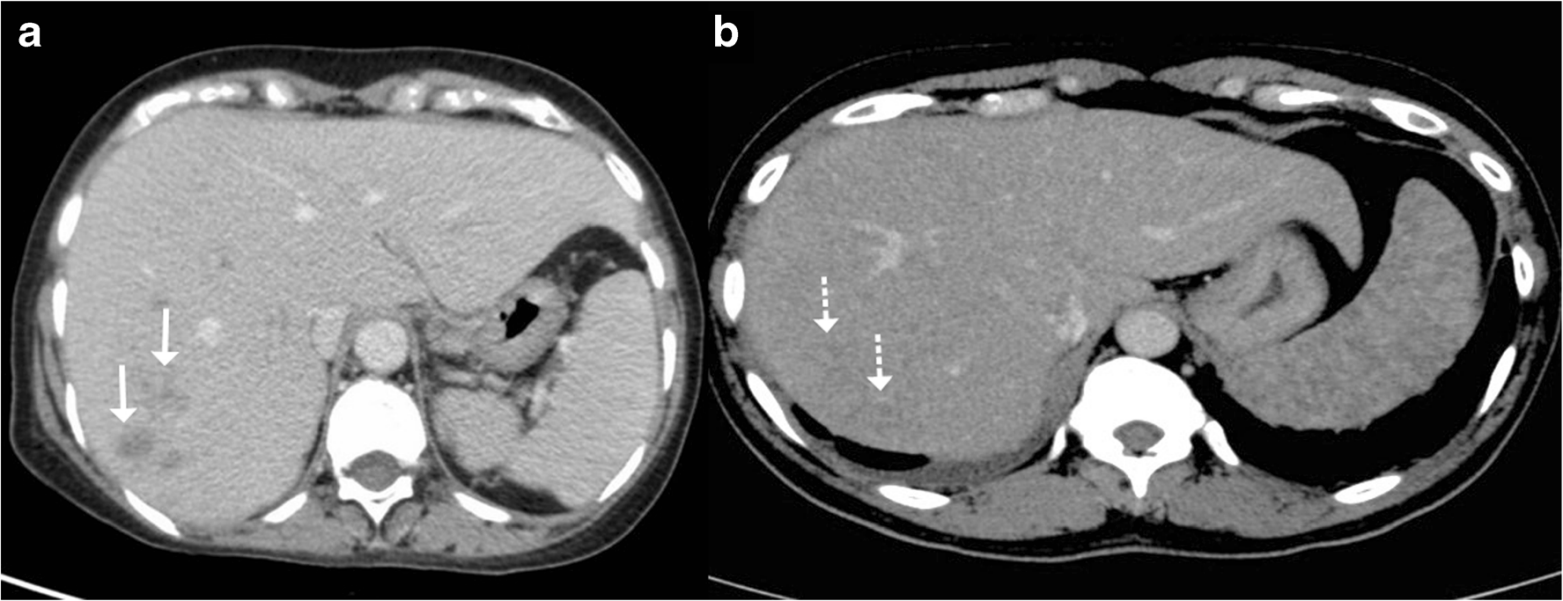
a Axial contrast-enhanced CT of a 55-year-old woman with fever and weight loss exhibits multiple hypodense lesions (*arrows*) in the right lobe of the liver. Biopsy from the lesions revealed null cell lymphoma. b CT images of a 40-year-old man with known sarcoidosis shows subtle hypodense hepatic lesions (*dashed arrows*) with heterogeneous appearance of the spleen
